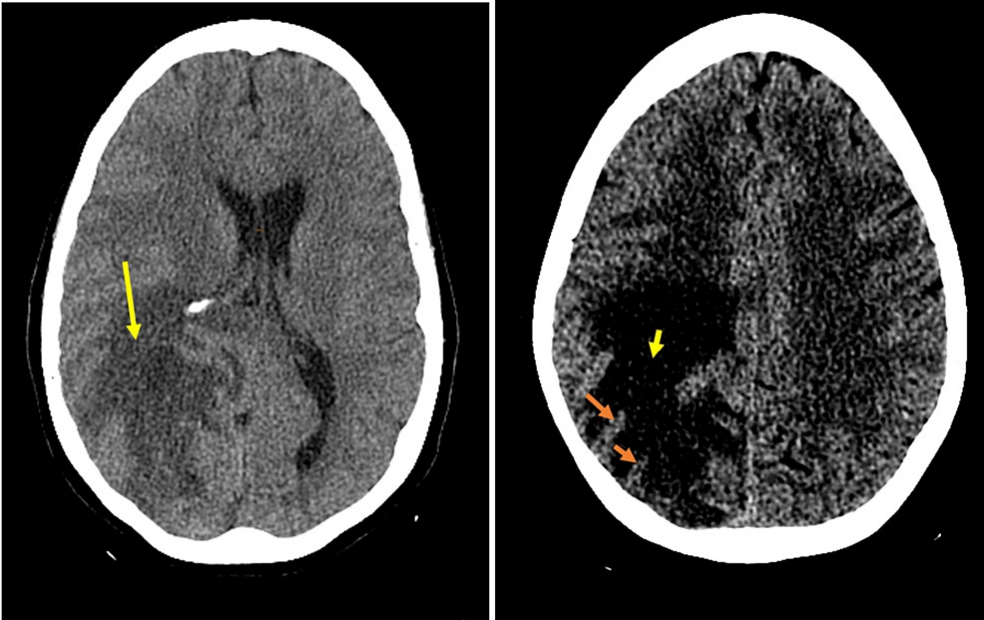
Initial CT scan of the head showed hypodensity within the right parietotemporal and occipital lobes with preservation of the gray-white differentiation [Orange arrows], consistent with vasogenic edema [Yellow arrow]. In addition, there is a mass effect with a midline shift of approximately 7 mm. These findings are concerning for the neoplastic process.
